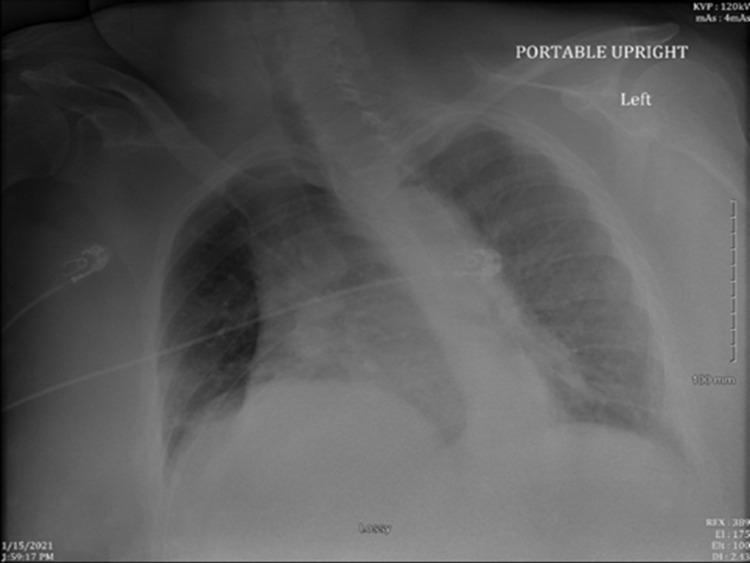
Chest X-ray with anteroposterior view revealing bilateral interstitial densities that suggest atelectasis or interstitial infiltrates.
